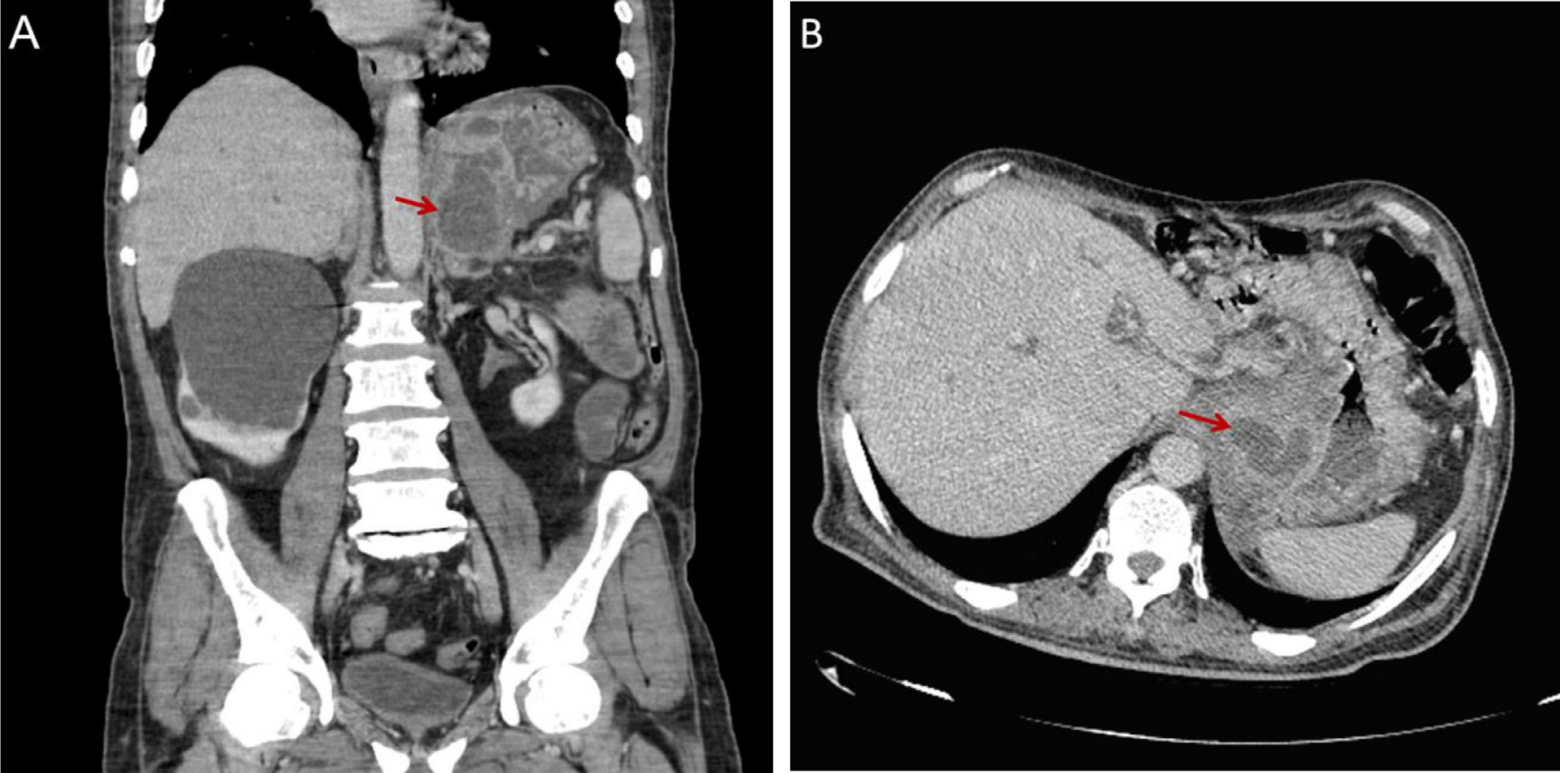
(A and B) Abdomen and pelvic CT scan, coronal and axial Views: A newly relatively thick-walled cystic structure measuring 65 × 53 mm is seen in the epigastric region, arising from the lesser curvature of the stomach, suggestive of an intramural gastric pseudocyst (red arrow). This is in addition to the 2 previously noted cystic structures in the greater curvature.
